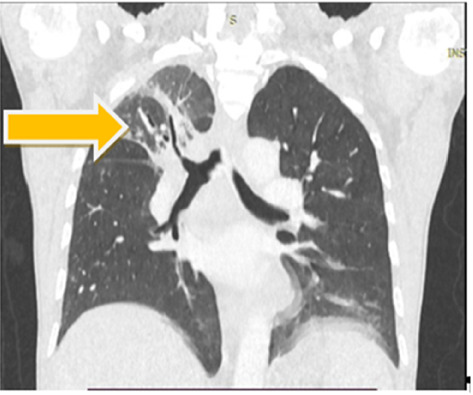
Cervicothoracic CT scan with and without contrast, frontal view showing tracheobronchial wall thickening with right apical atelectasis (yellow arrow).
